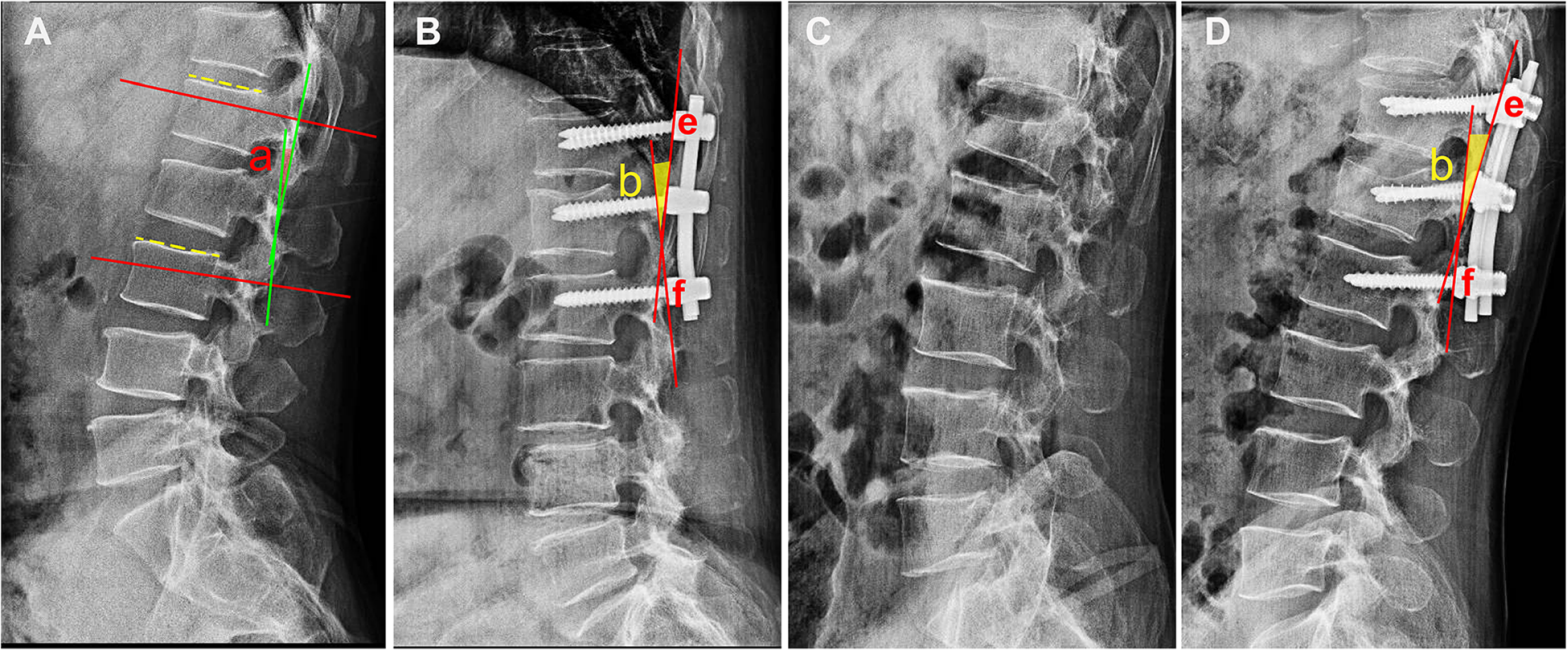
a The red lines indicate the parallel lines of the upper and lower endplates. The green lines indicate the vertical lines of the red lines. Angle a is the angle between the two green lines which is indicated as the sagittal Cobb* angle in the study. b The red lines indicate the tangents of the connecting points (point e and point f) of the rod and the upper and lower pedicle screws. Angle b is the angle between the two red lines which is indicated as the bending rod angle in this study. c The preoperative unstable thoracolumbar fracture lateral radiography. d The postoperative unstable thoracolumbar fracture lateral radiography. The red lines indicate the tangents of the connecting points (point e and point f) of the rod and the upper and lower pedicle screws. Angle b is the angle between the two red lines which is indicated as the bending rod angle in this study.
